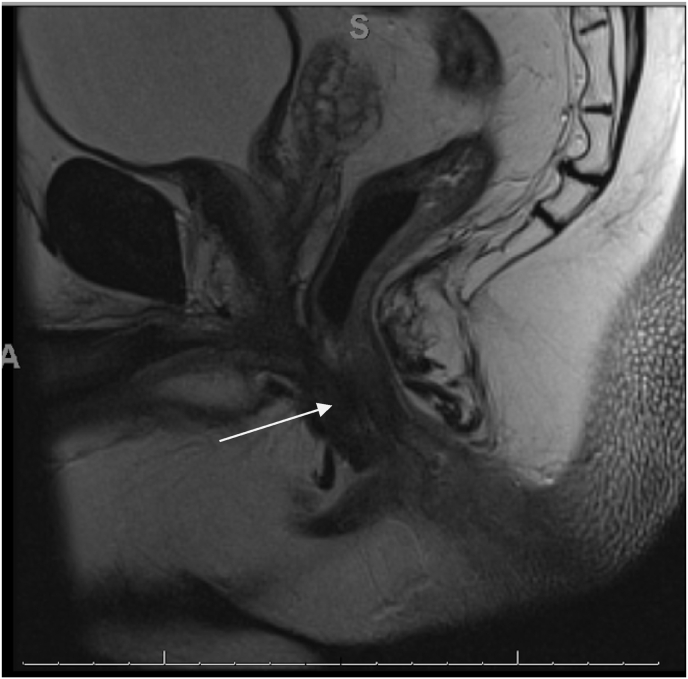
T2 MRI sagittal view of the mass shows invasion of the external sphincter complex.
